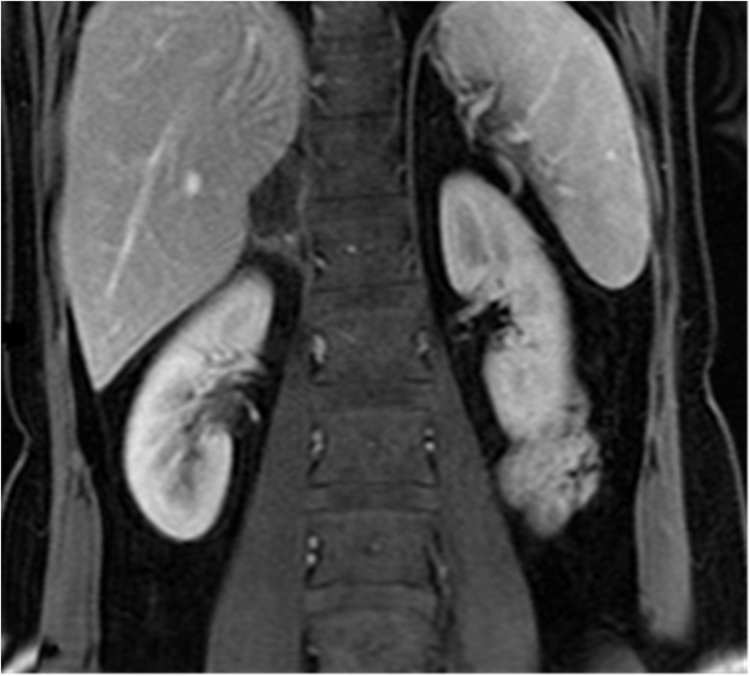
Close affiliation of the structures is shown through the first follow-up MRI, posterior to the injured adrenal gland seems not only compressed between the liver and the vertebrae, but also reshaped in contact with the latter.
